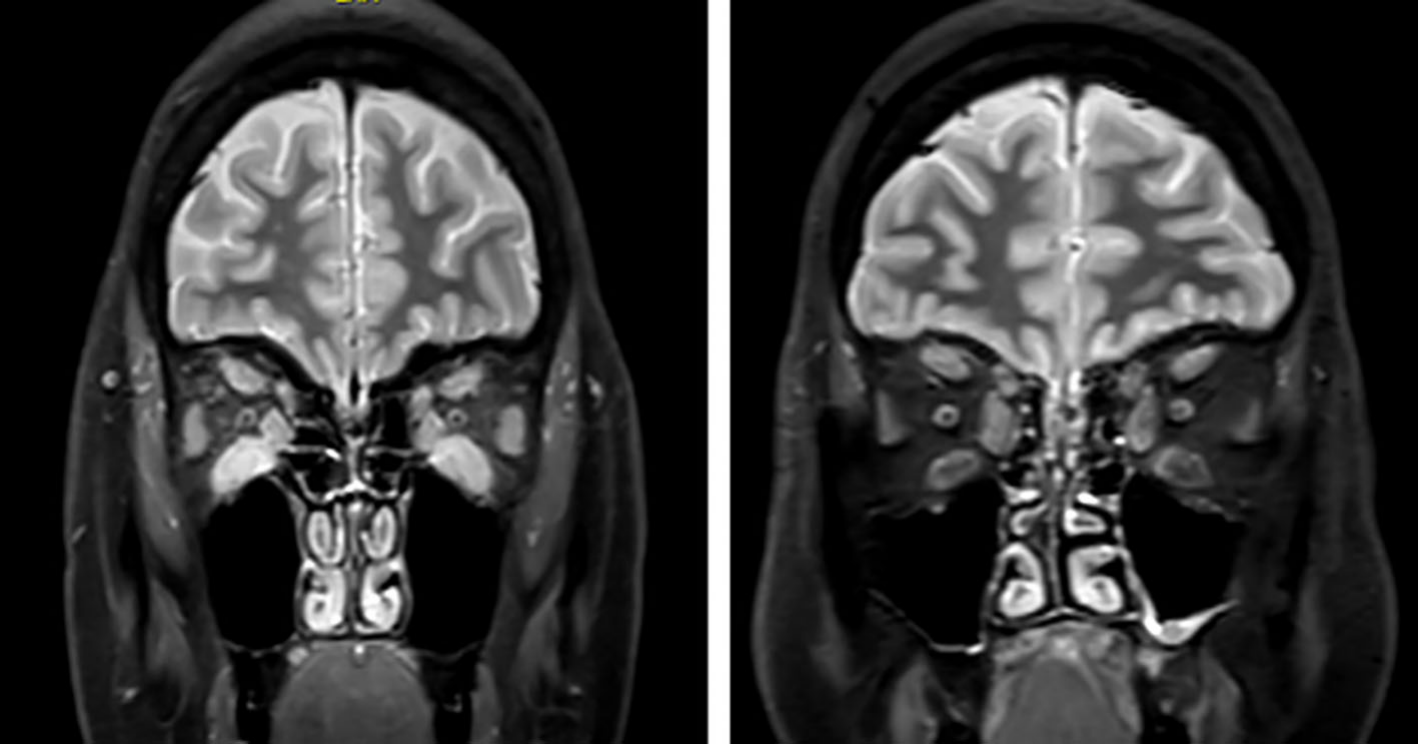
Left: coronal STIR MRI in a patient with active disease. Increased and infiltrated orbital fat tissue; thickening of all EOMs with edematous changes; no apical crowding. Right: same patient after intravenous glucocorticoid therapy. Proptosis decreased from severe to moderate on both sides clinically (not shown); normal fat tissue; fatty infiltration of EOMs with no edematous changes. STIR, short tau inversion recovery; EOMs, extraocular muscles.
